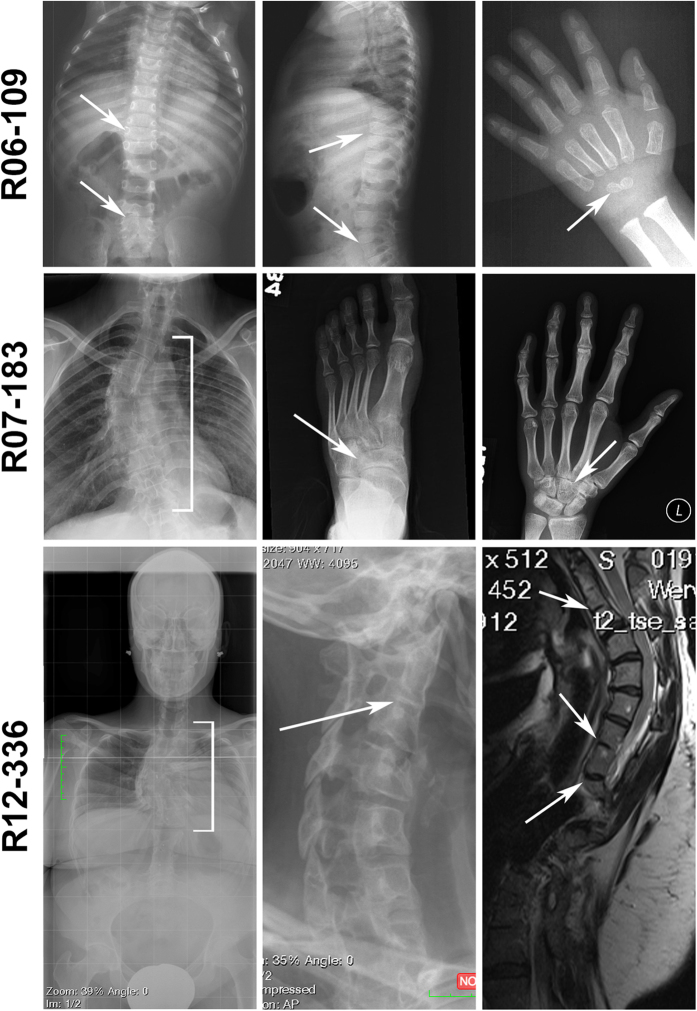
Radiographs of individuals with AD SCT. (R06-109) Three year old patient with SCT. *Left, Middle*: Radiographs of the spine showing narrowing disc spaces in the thoracic and lumbar spine (arrows). *Right*: Left hand showing developing carpal coalition (arrow). (R07-183) Adult patient with SCT. *Left*: Radiograph of the spine showing severe scoliosis and vertebral fusions in the thoracic spine (bracket). *Middle*: Left foot showing coalition of navicular and cuboid tarsal bones (arrow). *Right*: Left hand showing coalition of the hamate, trapezoid and trapezium carpal bones (arrow). (R12-336) Adult patient with SCT. *Left*: Full torso radiograph showing severe scoliosis and fusions in the thoracic spine (bracket). *Middle*: Cervical vertebrae showing narrowed disc space (arrow). *Right*: CT scan of the thoracic spine showing disc space obliteration and deformity (arrows).
